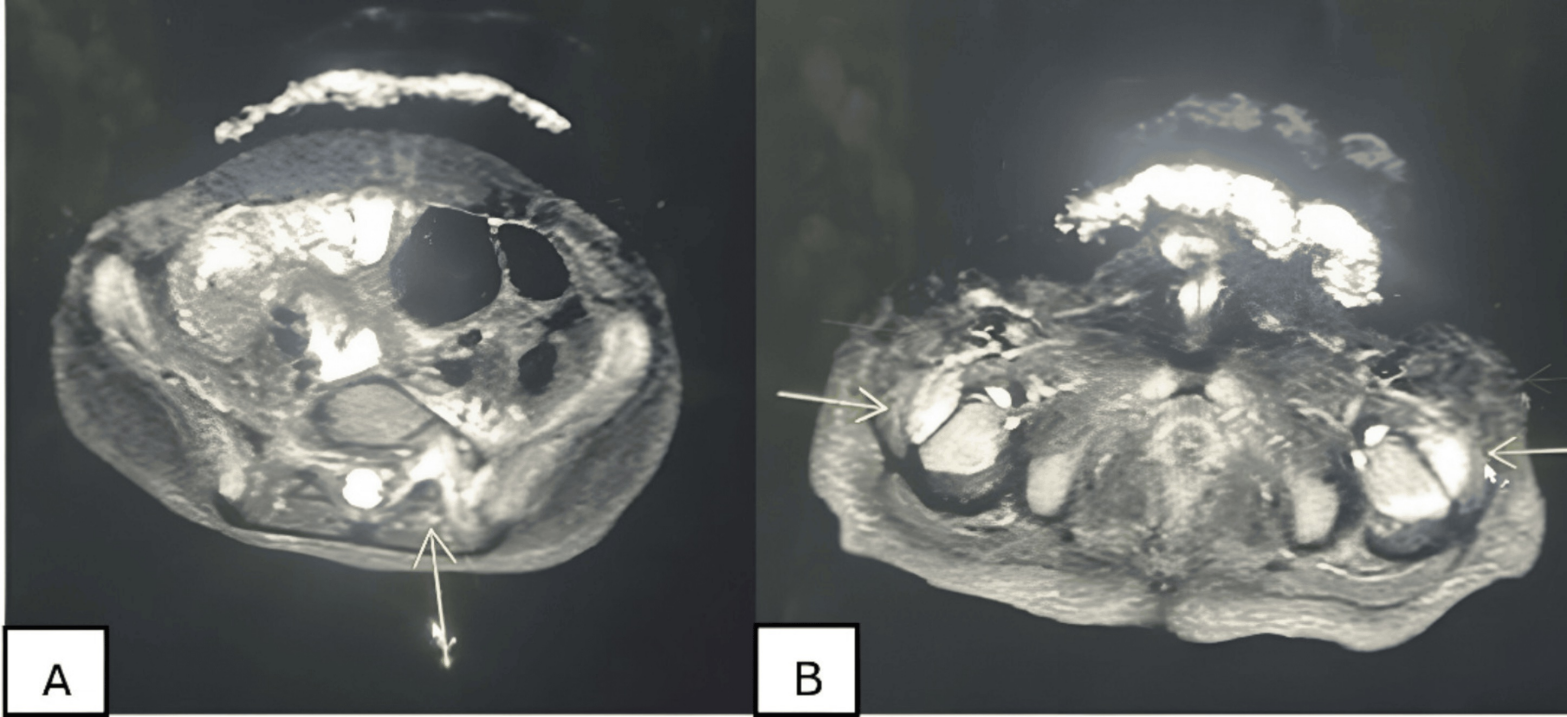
Full-body MRI showing septic arthritis of the left aspect of the L5 posterior elements (A) and iliac bones (B) with osteomyelitis (yellow arrows).
